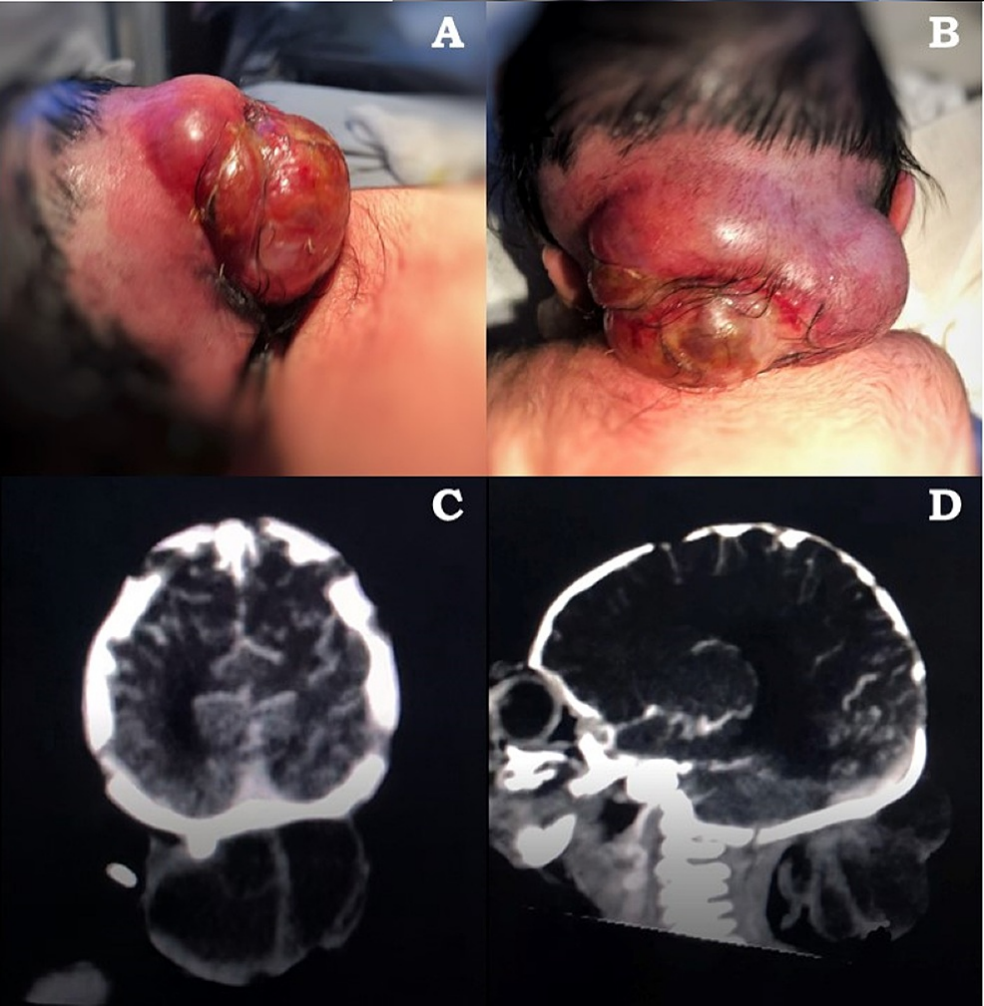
Occipital encephalocele (A) Occipital encephalocele lateral view, (B) occipital encephalocele superior view, (C) axial head CT showing the cystic protrusion consistent with occipital encephalocele, and (D) sagittal head CT image showing the same cystic protrusion with heterogeneous densities.
